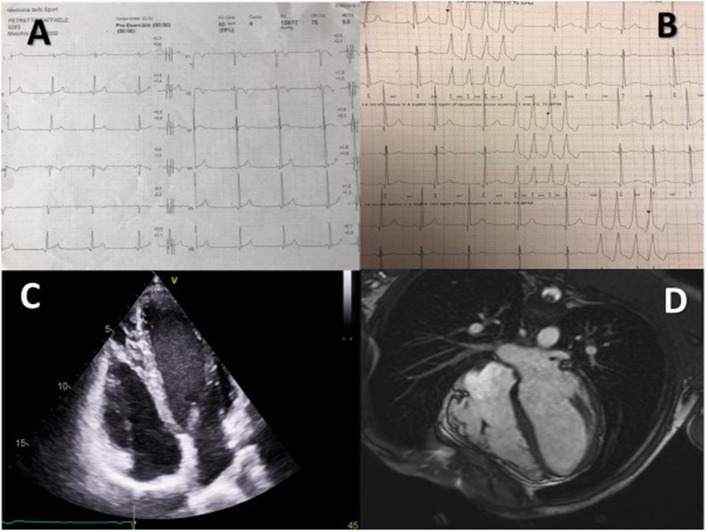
A 15 year-old male proband affected by AC, considered for an S-ICD. Table A shows typical T wave inversion in V1–V2 and diphasic T wave in V3, B shows recurrent NSVTs, C shows structural alterations in the right ventricle and D shows dysmorphic and diskinetic aspects of the right ventricle in the cardiac MRI.
